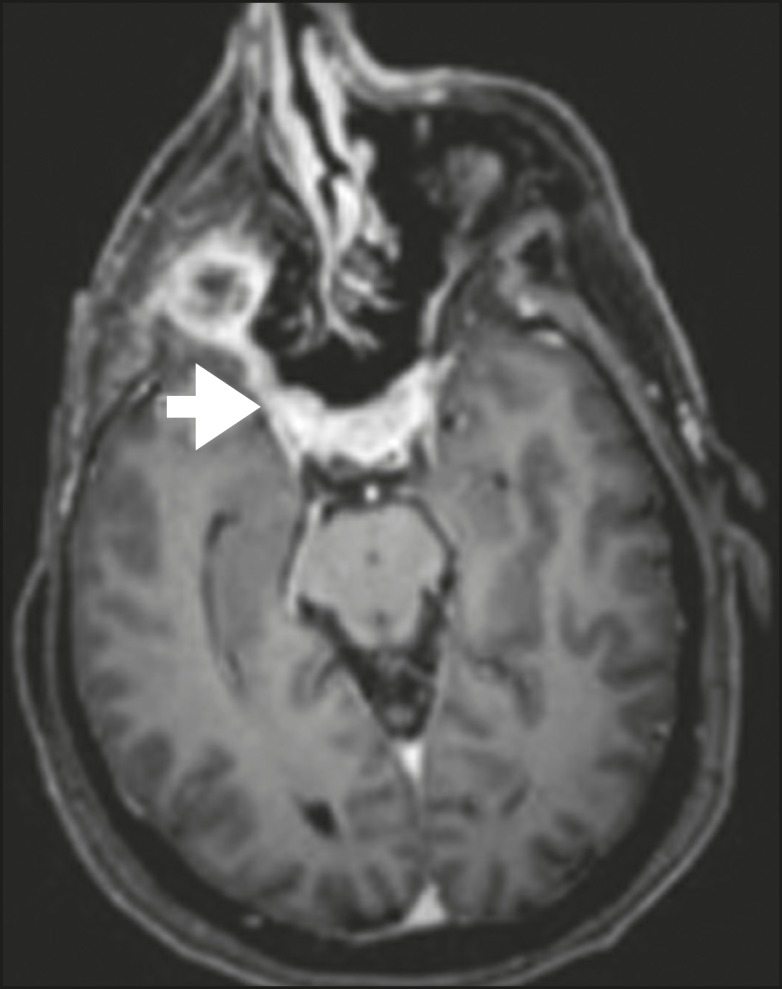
T1-weighted MRI scan of a 59-year-old male with (postoperative) squamous
